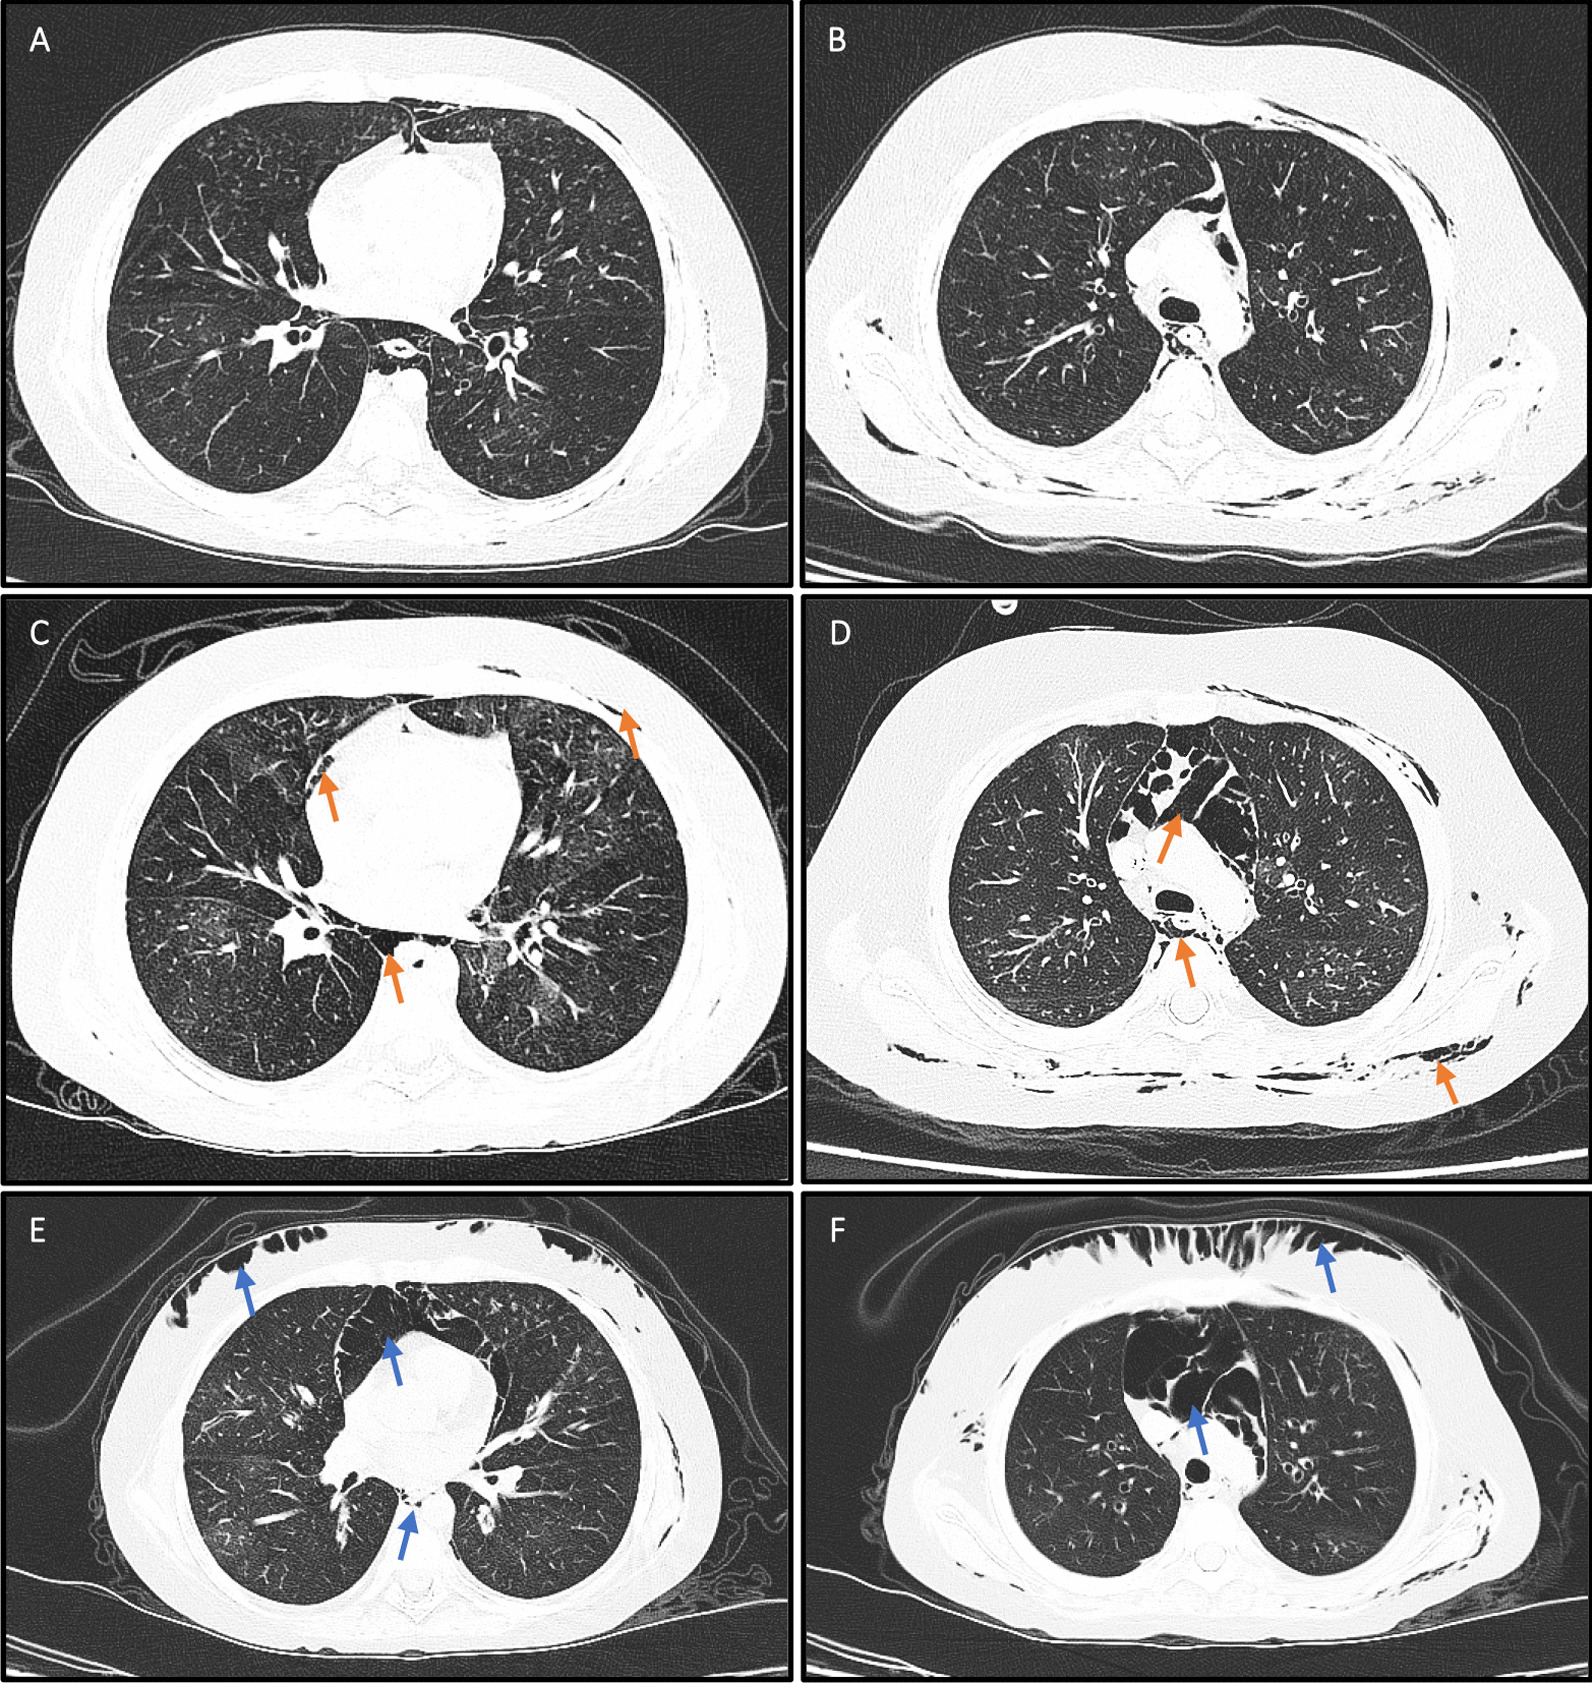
Chest CT images of the third case, who developed COVID-19 infection four months after AT-MSC therapy (panels A and B). Two weeks later, pneumomediastinum, pneumopericardium, and subcutaneous emphysema (*orange arrows*) progressed (panels C and D); however, these abnormalities did not show a significant progression (*blue arrows*) in images obtained 12 months later (panels E and F)
